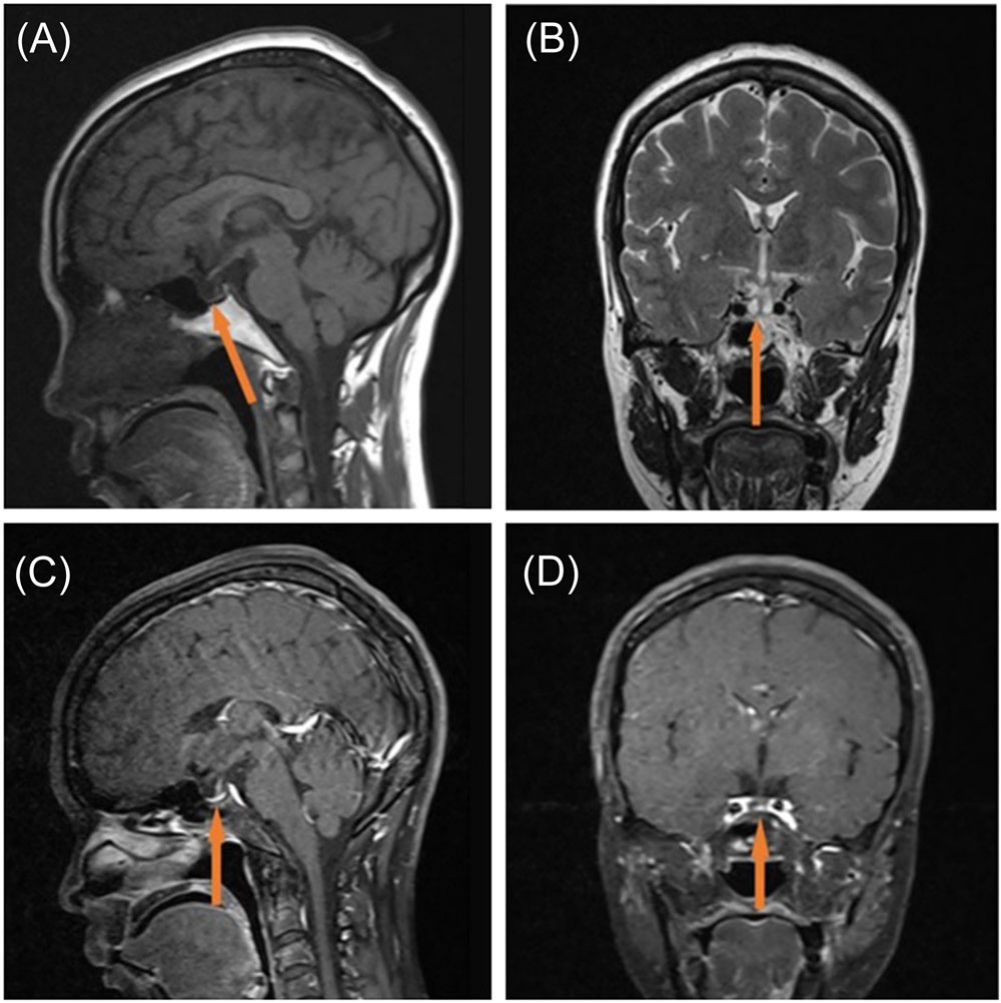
Nuclear magnetic resonance imaging manifestations of the patient indicate pituitary atrophy (orange bold arrow): (A) sagitta T1 weighted, (B) coronal T2 weighted, (C) sagitta T1‐weighted postcontrast, and (D) coronal T1‐weighted coronal. [Color figure can be viewed at wileyonlinelibrary.com]
